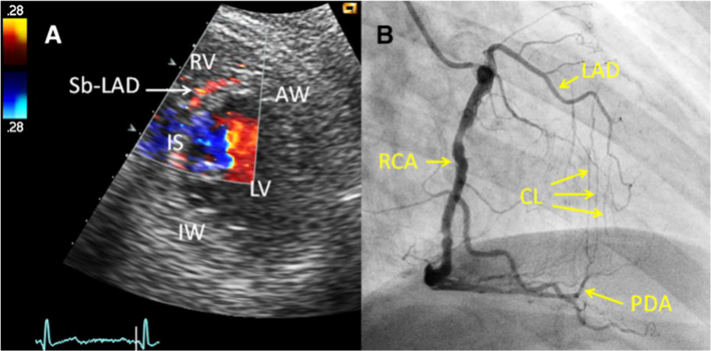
Example of a transthoracic Doppler finding of retrograde collateral blood flow in a septal perforating branch, compared with coronary angiography. (A) In a modified parasternal short-axis view, a septal perforating branch from the left anterior descending coronary artery (Sb-LAD) is seen with retrograde blood flow. (B) Angiogram of the right coronary artery (RCA) demonstrates collateral (CL) blood supply from the posterior descending coronary artery (PDA) through the septal perforating branches to the left anterior descending coronary artery (LAD). Angiogram of the left coronary artery demonstrated proximal LAD occlusion (not shown). AW = anterior wall of the left ventricle, IS = interventricular septum, IW = inferior wall of the left ventricle, LV = left ventricle, RV = right ventricle.
